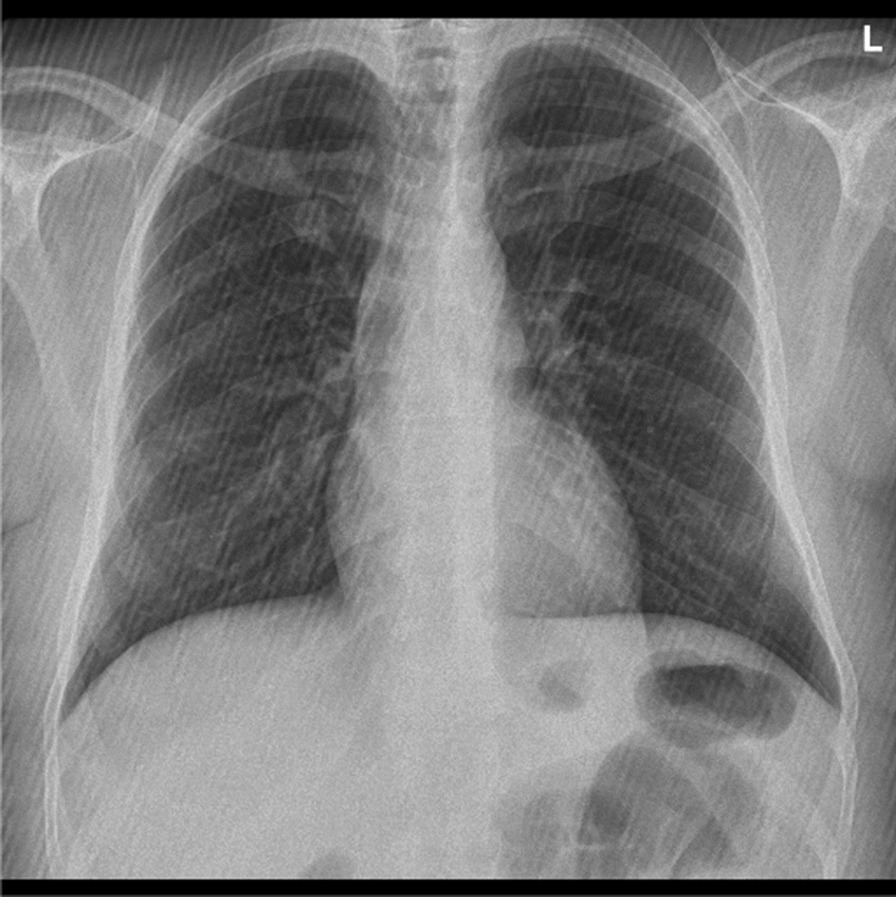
Showing the normal chest x-ray
